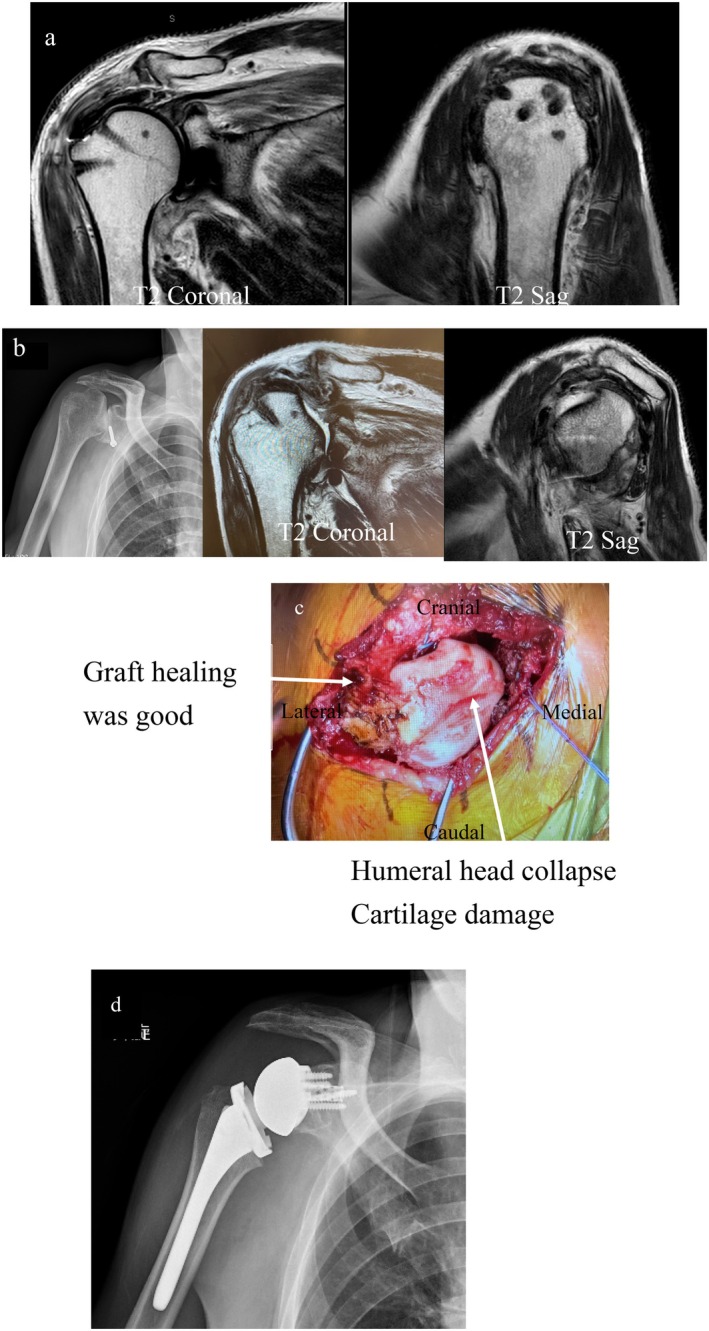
Postoperative course and RTSA. (a) MRI at 3 months confirming intact graft. (b) Radiograph at 7 months showing humeral head collapse (Cruess stage IV). (c) Intraoperative photo demonstrating humeral head collapse with preserved graft incorporation. (d) Postoperative radiograph after reverse total shoulder arthroplasty (RTSA).
